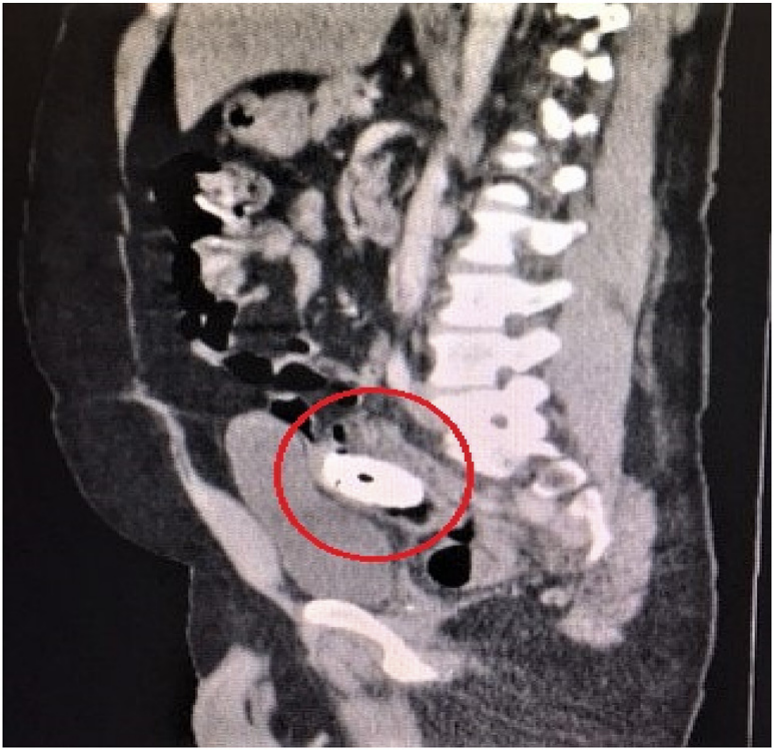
CT sagittal view image depicting the foreign body trajectory, circled in red.
